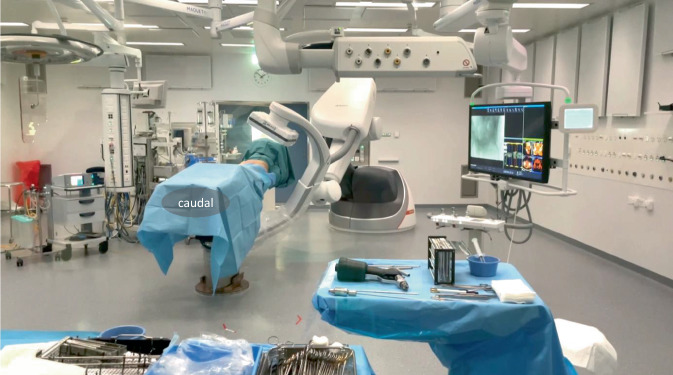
The first step is performing a 3D scan for matching with the diagnostic CT scan for abbreviations and further explanations see *appendix* *3*. The staff leaves the room during acquisition of the 3D scan to reduce radiation exposure. Matching consists of manual approximation, but final matching is done by the computer
